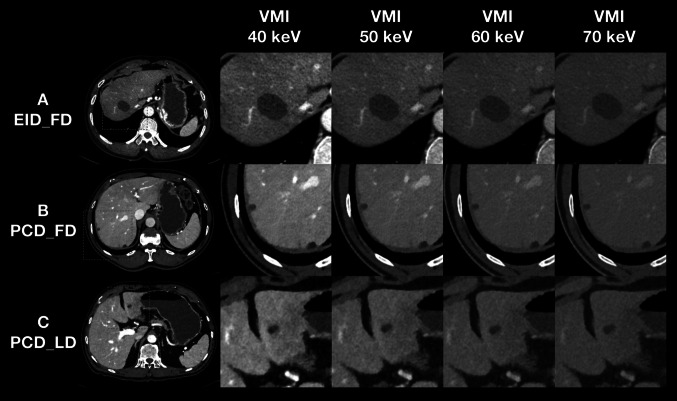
Representative cases for hepatic lesion conspicuity assessment. Three matched cases of EID_FD, PCD_FD, PCD_LD scans of VMI at 40, 50, 60, and 70 keV for hepatic lesion assessment are presented with a window width of 800 HU and window level of 200 HU. (A) A 59-year-old male patient with a height of 1.73 m, a weight of 72.0 kg, and a body mass index of 24.05 kg/m2, who scheduled to undergo the contrast-enhanced abdominal CT scan for heartburn and acid regurgitation, on EID-CT with a full-dose protocol. (B) A 61-year-old male patient with a height of 1.82 m, a weight of 79.0 kg, and a body mass index of 23.85 kg/m^2^, who scheduled to undergo the contrast-enhanced abdominal CT scan for abdominal pain, on PCD-CT with a full-dose protocol. (C) A 60-year-old male patient with a height of 1.72 m, a weight of 71.0 kg, and a body mass index of 24.00 kg/m^2^, who scheduled to undergo the contrast-enhanced abdominal CT scan for abdominal discomfort, on PCD-CT with a low-dose protocol. The contrast between the hypodense hepatic lesion and liver parenchyma and the border of the lesion became clearer with the decreasing of keV level of VMI, but the image noise got worse and potentially impacted on the lesion conspicuity. The lesion conspicuity of PCD_FD and PCD_LD benefited from the better overall image quality compared to EID_FD. EID_FD = energy-integrating detector CT with full-dose protocol; PCD_FD = photon-counting detector CT with full-dose protocol; PCD_LD = photon-counting detector CT with low-dose protocol, VMI = virtual monoenergetic imaging, keV = kiloelectron volt
